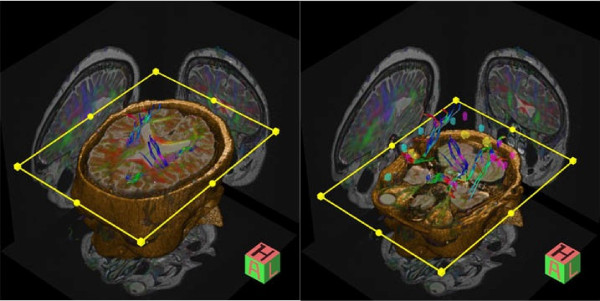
Magnetic resonance imaging of our patient. The transverse image is unremarkable and does not indicate minor traumatic brain injury. This is the same plane where diffusion tension imaging indicates trauma.
